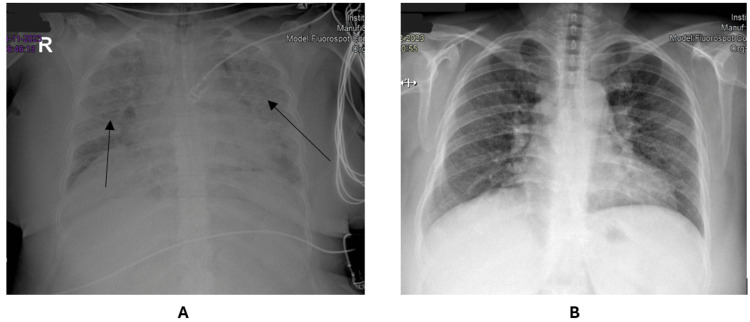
Chest X-ray at admission (A) and at discharge (B)
